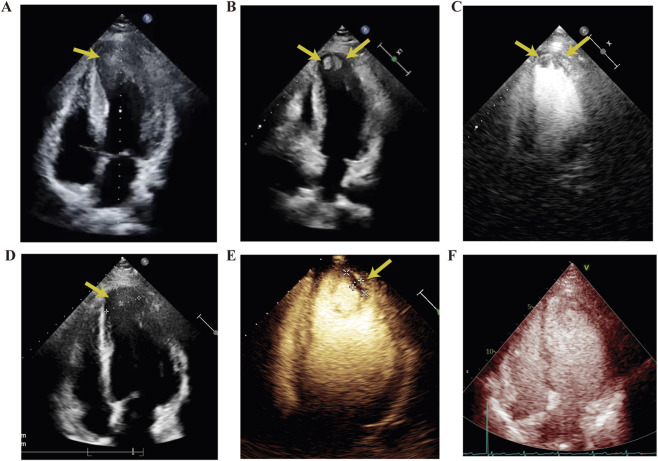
Serial echocardiographic monitoring of left ventricular thrombus. (A) Pre-PCI transthoracic echocardiography showing apical hypokinesia with vortex-like echoes indicating blood stasis. (B) Echocardiography immediately after stroke onset revealed two mobile mass-like thrombi in the left ventricular apex (1.6 × 1.1 cm and 1.3 × 1.1 cm, arrows). (C) One-week follow-up contrast-enhanced TTE showing persistent apical thrombus despite rivaroxaban therapy (arrow). (D) Routine TTE at 1 month revealing an apical ventricular aneurysm (3.0 × 1.5 cm, arrow) without significant thrombus. (E) Contrast-enhanced TTE at 1 month demonstrating residual thrombus (1.8 × 0.7 cm, arrow) with reduced size and mobility. (F) Six-month contrast-enhanced TTE confirming complete resolution of left ventricular thrombus.
